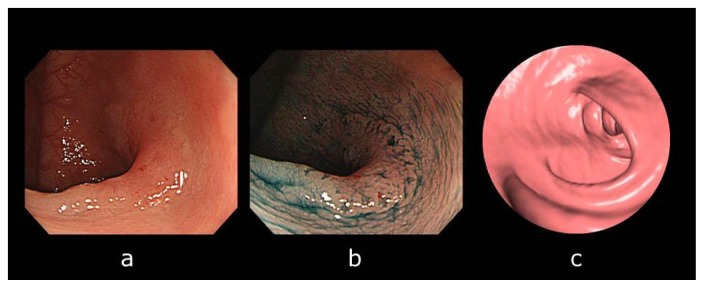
A laterally spreading tumor (LST) not observed by computed tomographic colonography (CTC) in a 44-year-old man. (a) Colonoscopy revealed an IIa + IIc (LST non-granular type: LST-NG) lesions, 25 mm in diameter located in the transverse colon; (b) The lesion margin became apparent after indigo-carmine dye spraying; and (c) We could not observe the lesion by means of CTC at the time of the analysis even when we knew the results of the colonoscopy. Endoscopic submucosal dissection (ESD) was subsequently performed on this LST-NG lesion. Pathological diagnosis was a well-differentiated adenocarcinoma with an sm_2_ invasion depth of 1500 μm from the musclaris mucosae. Because the invasion depth was sm_2_, an additional surgery was performed.
